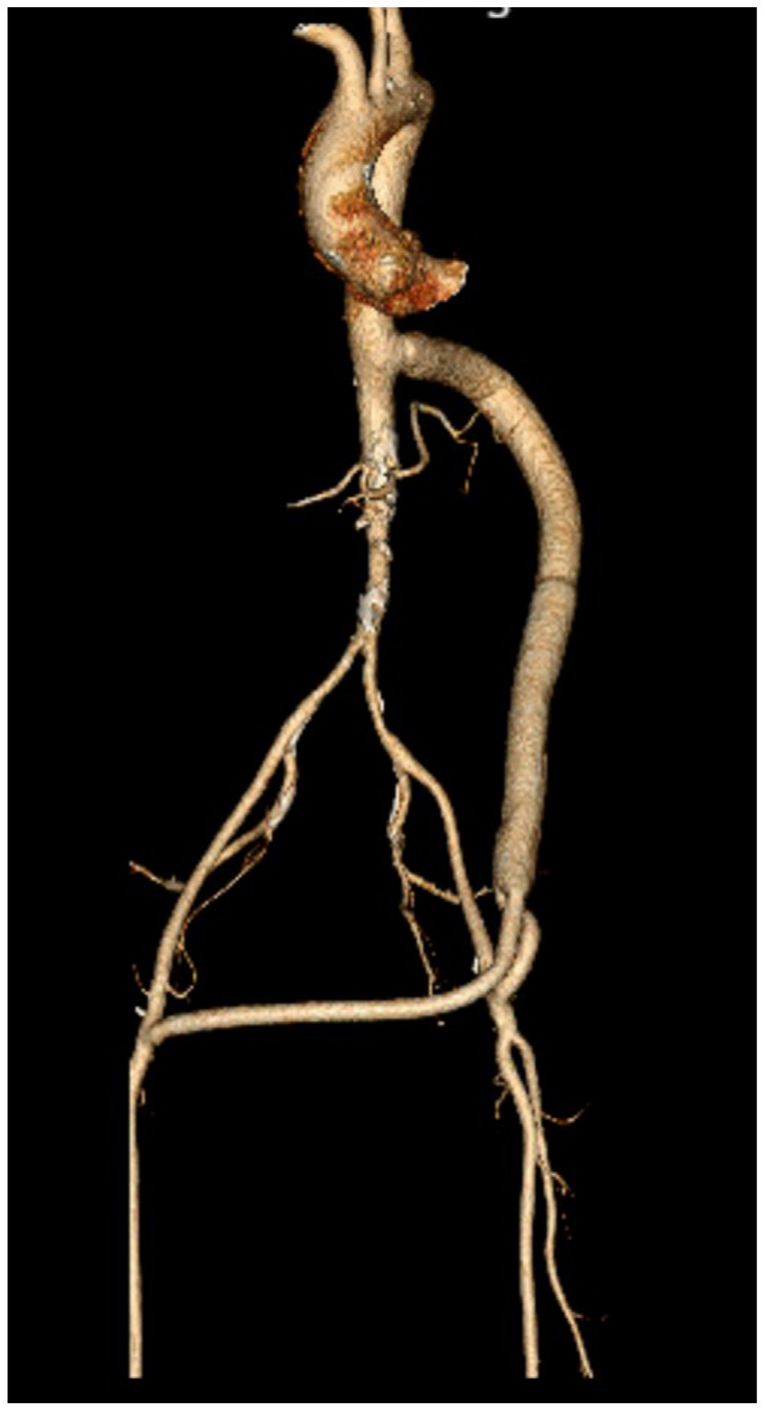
Three-dimensional computed tomography reconstruction showing a thoracobifemoral bypass (TBF) graft originating from the descending thoracic aorta and extending to the bilateral common femoral arteries (CFAs).
